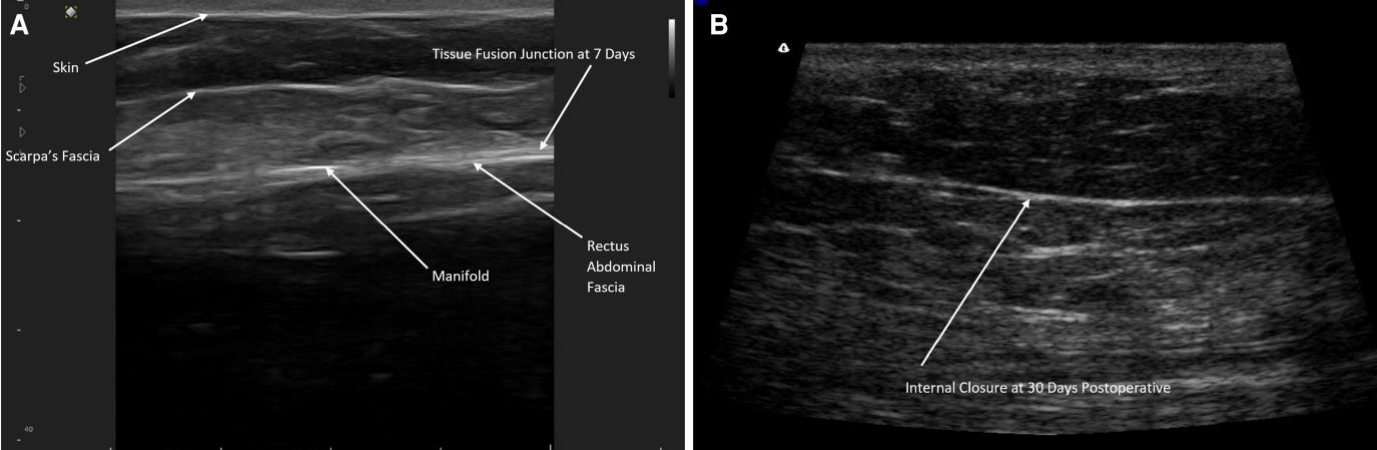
Formal documentation of the plane of tissue closure with overlying abdominal flap healing to the underlying fascia with no fluid collection present. Internal tissue closure on high-resolution ultrasound (HRUS) shown on (A) postoperative Day 7 and (B) postoperative Day 30.
